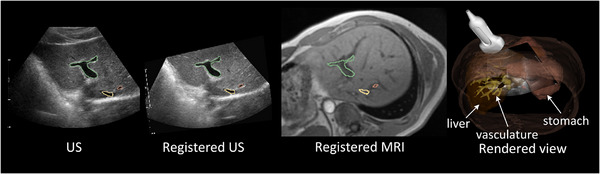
Registration result overview of 3 test cases. Segmented vessels in the 3D US image are overlaid on the 2D US image and registered with MRI images, respectively.
